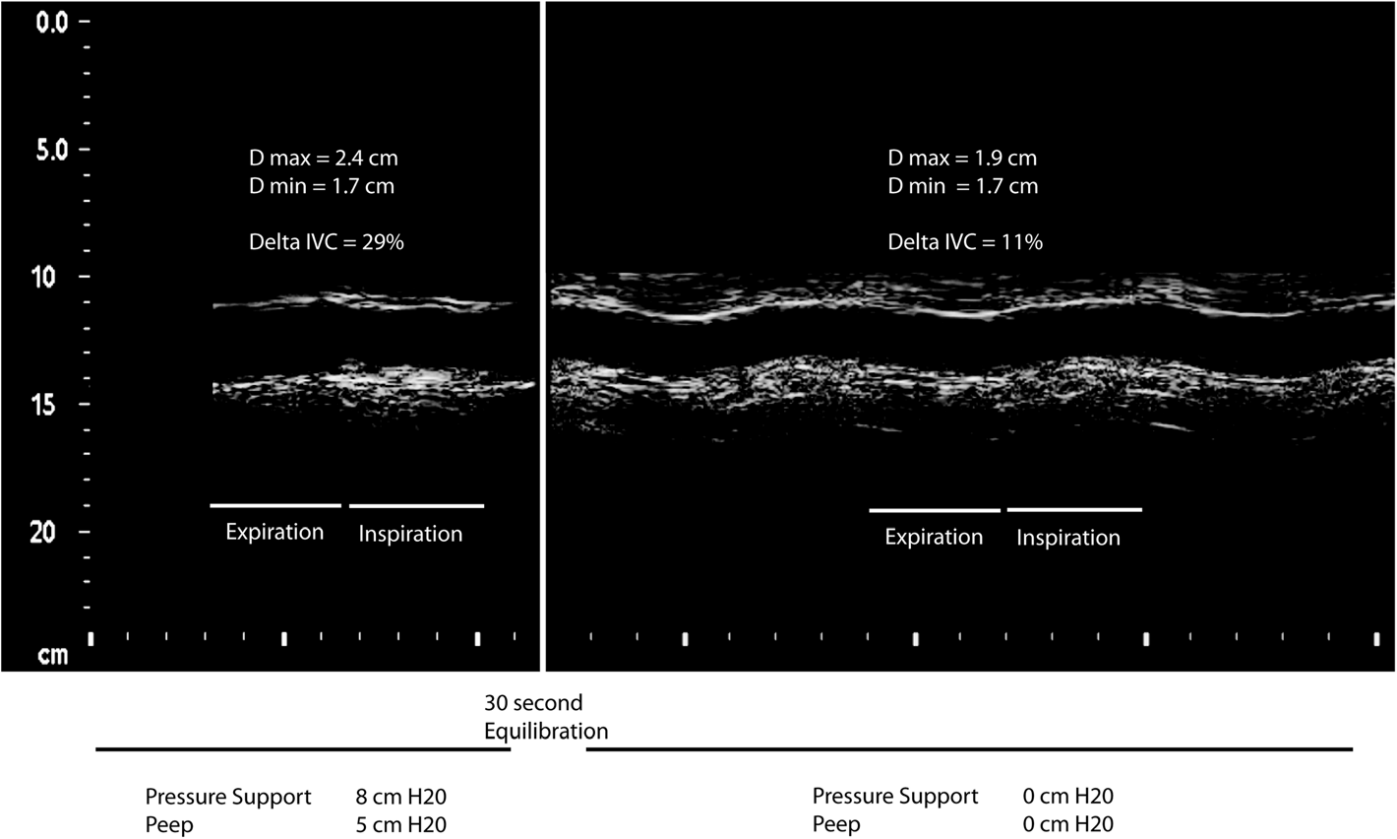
A 57-year-old male patient admitted with septic shock 18 hours before imaging required 0.2 μg/kg/minute of norepinephrine to maintain a mean arterial blood pressure of 70 mmHg. Central venous pressure via the right internal jugular catheter was 13 mmHg and he was in atrial fibrillation, rate of 100 beats/minute. Sedation had been discontinued and the patient was awake and spontaneously breathing on a mechanical ventilator. Using a subcostal approach the IVC was imaged using M-mode at 1.5 cm from the IVC–right atrial junction. The patient then began a spontaneous breathing trial, with some translational movement of the IVC noted, and imaging continued. In this case the IVC diameter during inspiration did not change according to the level of pressure support, whereas the end-expiratory IVC diameters were markedly greater with positive pressure applied. Thus the delta IVC during usual mechanical ventilation was 29 %, while during his spontaneous breathing trial the delta IVC was only 11 %. A CardioQ™ esophageal Doppler probe was in place and an optimal descending aortic blood flow was calculated. In this patient the stroke volume increased from 49 to 65 ml (33 % increase) with a 500 ml bolus of plasmalyte™, and thus was truly volume responsive. *IVC* inferior vena cava, *PEEP* positive end-expiratory pressure
